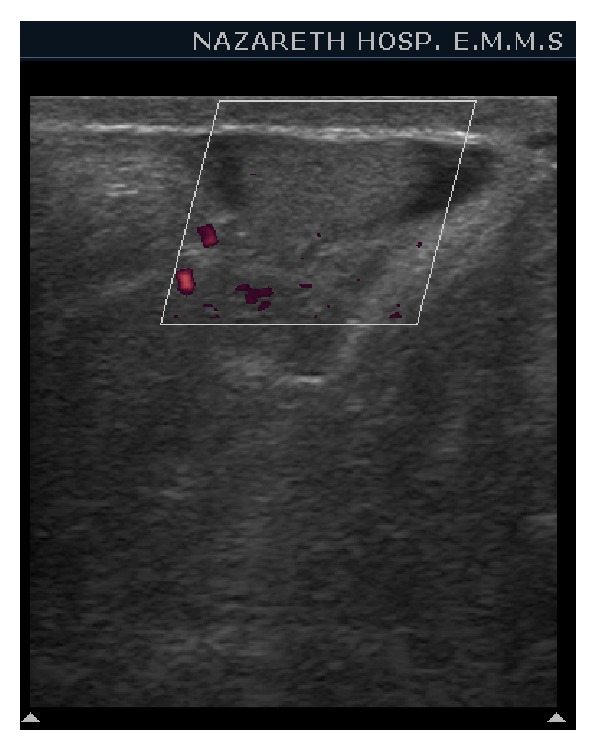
Ultrasound color Doppler of the inguinal area shows an inguinal oval 1.6 cm, hypoechogenic mass with no flow in it that resembles small testis.
